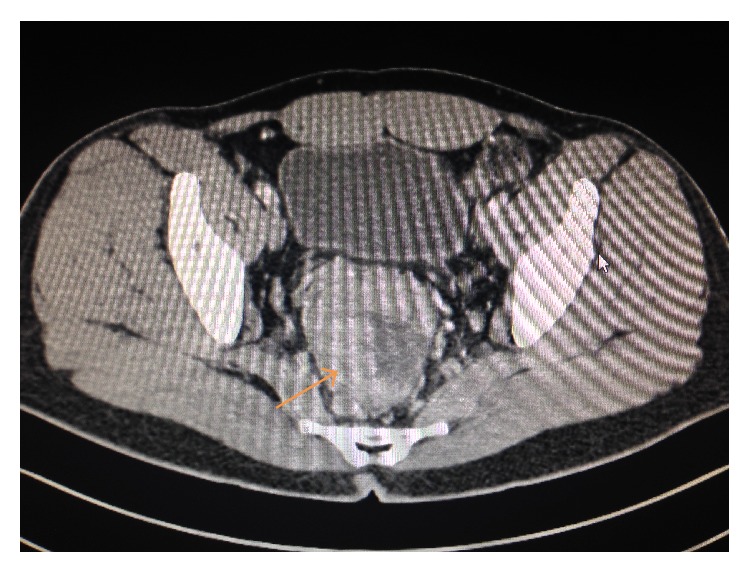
Abdominal CT scan showing large exophytic enhancing mass in the rectosigmoid colon with diffuse wall thickening.
